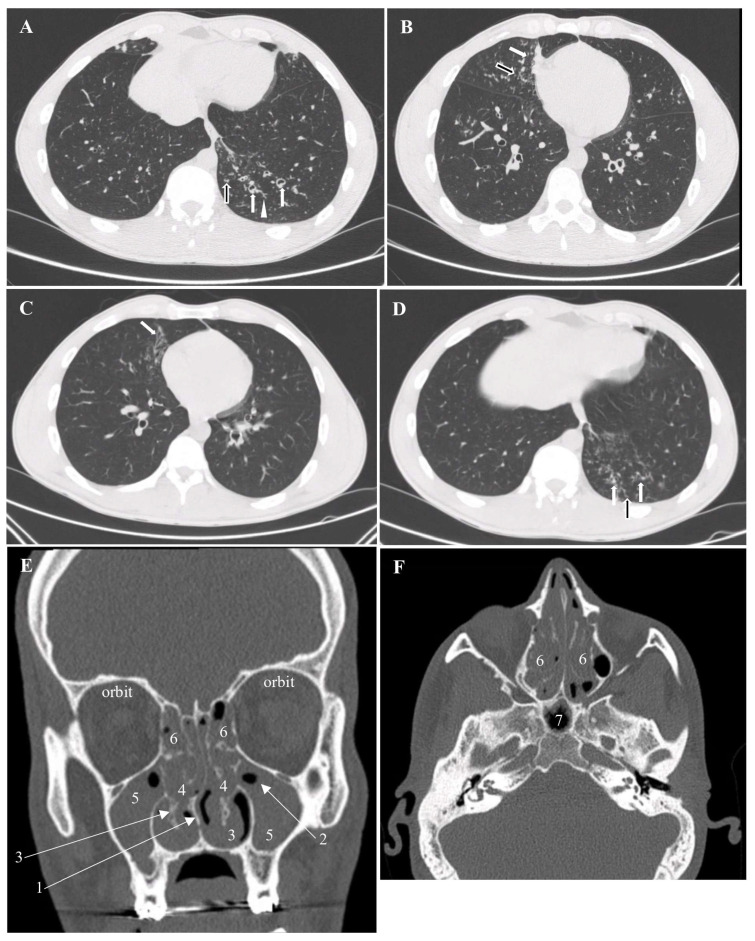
Computed tomography (CT) scan of the chest and paranasal sinuses in the patient. (A) Axial view of chest CT scan performed in February 2023, showing the presence of bronchiectasis (white arrows), micronodules (black arrow), peribronchovascular thickening, and tree in bud (white arrowhead) in the lung’s left lower lobe. (B) Axial view of chest CT scan performed in February 2023, showing the presence of bronchiectasis (black arrow), micronodules, peribronchovascular thickening, and tree in bud (white arrow) in the lung’s middle lobe. (C) Axial view of chest CT scan performed in August 2023, showing the presence of bronchiectasis and peribronchovascular thickening (white arrow) in the lung’s middle lobe. (D) Axial view of chest CT scan performed in August 2023, showing the presence of bronchiectasis (white arrows), micronodules, peribronchovascular thickening, and tree in bud (black arrow) in the lung’s left lower lobe. (E,F) Coronal (E) and axial (F) views of paranasal sinuses CT scan of the patient performed in February 2023, showing nasal polyposis and inflammatory pansinus disease; blockage of the ostiomeatal complexes; dextroconvex deviation of the nasal septum with a right parasagittal bone spur; inferior turbinate hypertrophy; partial obliteration of the nasal fossae; hypertrophy of the nasopharyngeal lymphatic tissue; sclerodiploic mastoids; and inflammatory filling of the remaining mastoid cells and the right tympanic cavity. (1) Nasal septum, (2) ostiomeatal complex, (3) inferior turbinate, (4) middle turbinate, (5) maxillary sinus, (6) ethmoid sinus, and (7) sphenoid sinus.
